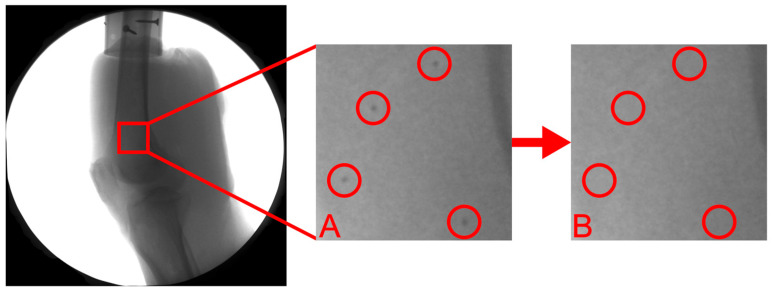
Example of bead masking: (A) region of BVR radiograph with beads visible, circled in red; (B) identical region after the bead masking algorithm was applied. NB: The image contrast of (A,B) was enhanced to improve visibility of beads for demonstrative purposes only.
